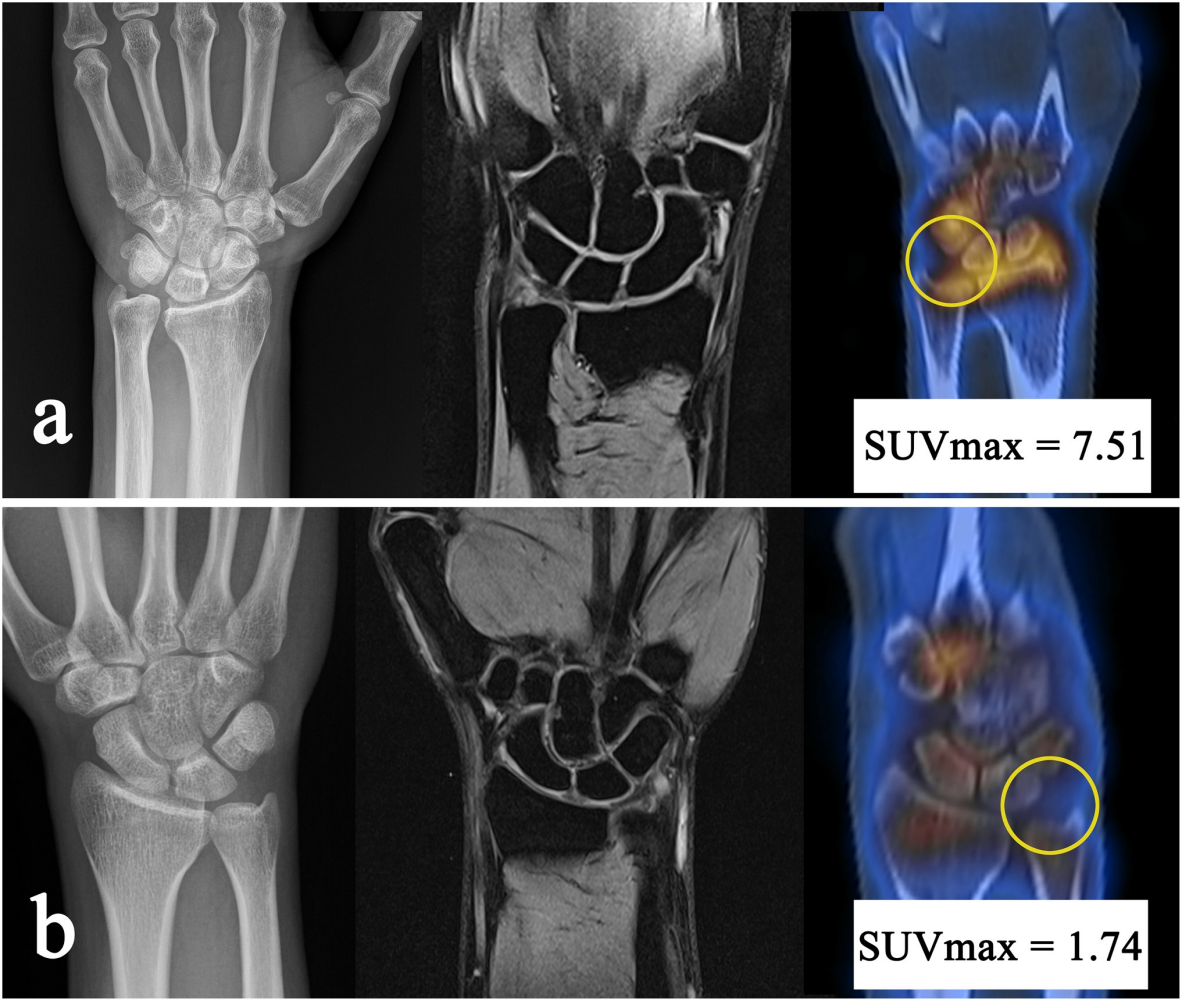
Examples of clinical applications of the maximum standard uptake values (SUVmax). (a) Images of a 47-year-old man. Plain radiography shows a positive ulnar variance. Magnetic resonance imaging (MRI) suggests central and foveal tear of the triangular fibrocartilage complex (TFCC), but there is no evidence of bone bruises or cystic change of the lunate. The semiquantitative SPECT/CT shows a 7.51 SUVmax for the ulnar-sided wrist, indicating active ulnar impaction syndrome (UIS). This patient could be diagnosed with UIS. (b) Images of a 22-year-old man. Plain radiography shows a positive ulnar variance. TFCC of the MRI suggested tear of the ulnar styloid and foveal attachment. There were no typical findings of the UIS between the carpal bones and distal ulna. The semiquantitative SPECT/CT shows mild uptake of the ulnar-side wrist and SUVmax of 1.74 for the ulnar-sided wrist that is lower than the cutoff value of 4.09 for UIS. This patient could be diagnosed with a TFCC tear without UIS.
